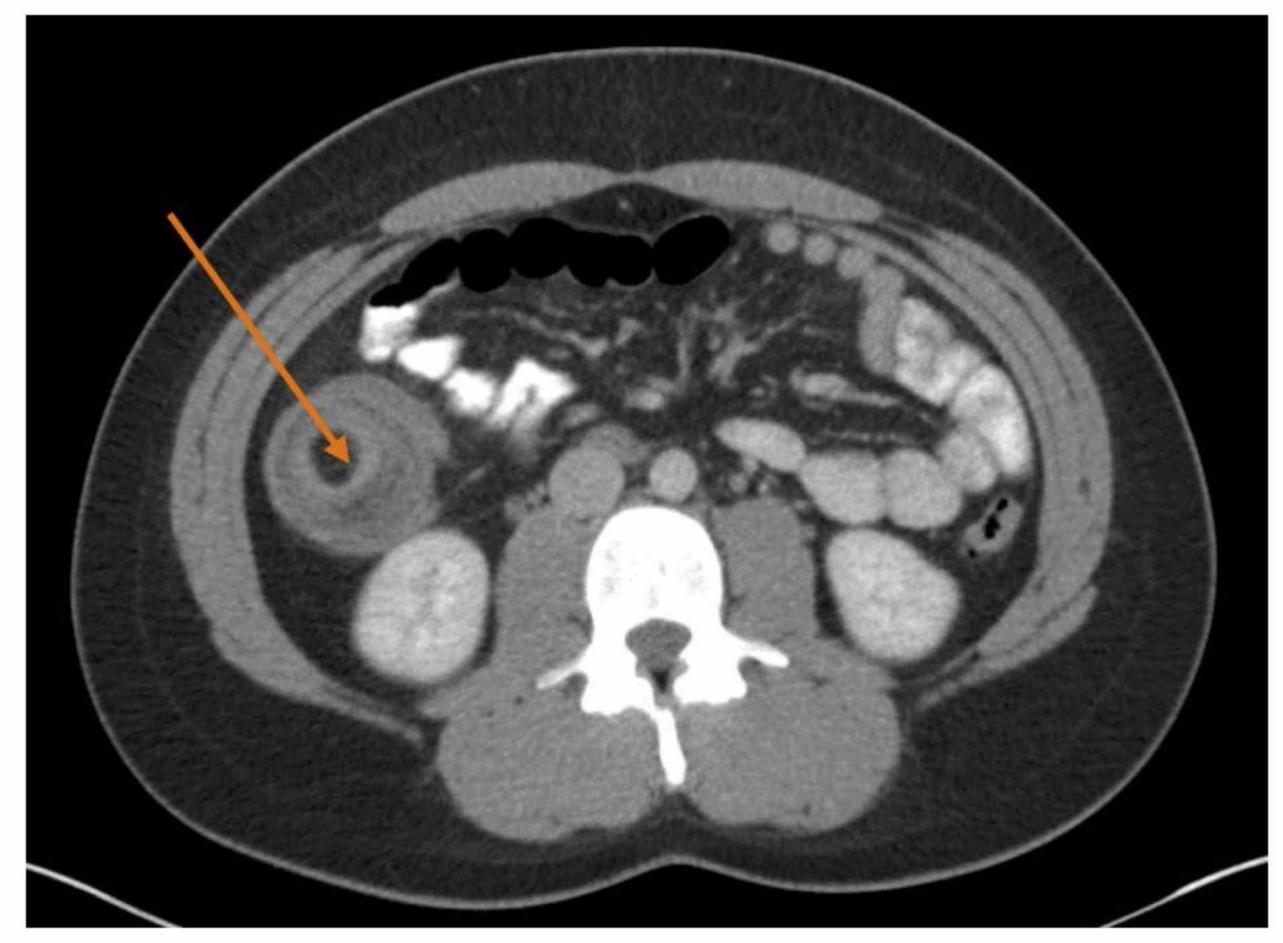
Computed tomography of abdomen and pelvis with contrast The transverse view of computed tomography (CT) of abdomen and pelvis with contrast. An ileocolic intussusception is present (orange arrow) with the terminal ileum telescoping into the ascending colon over an 8-centimeter interval.
